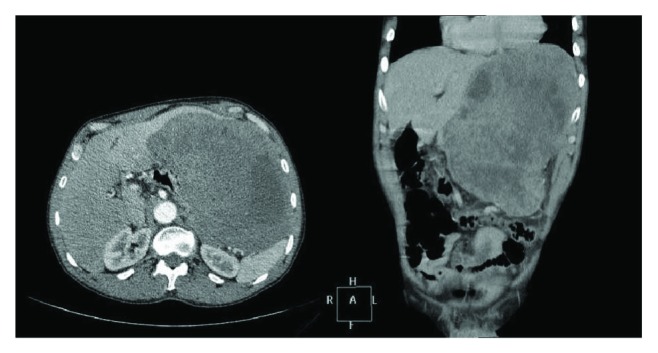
Contrast-enhanced computed tomography showing wide hypodense mass in the left hemiabdomen.
